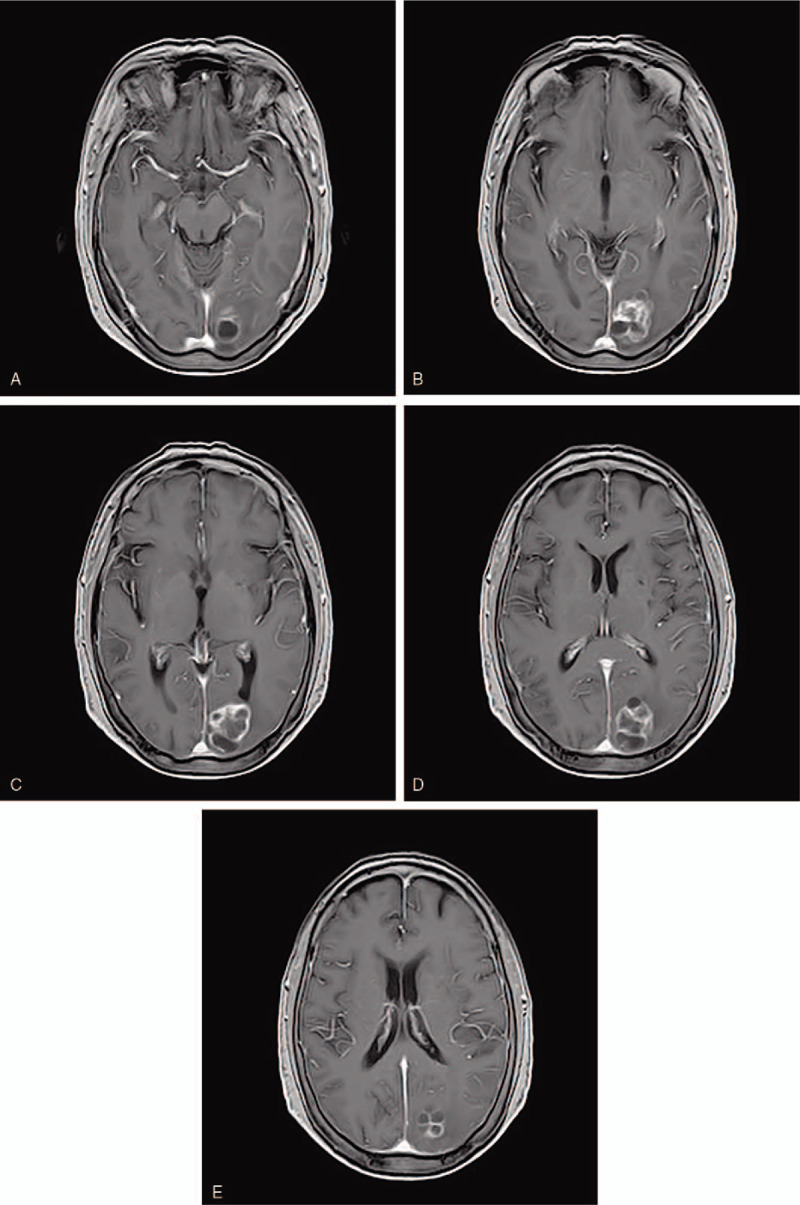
(A–E) Magnetic resonance imaging of left occipital metastasis at the end of radiotherapy.
